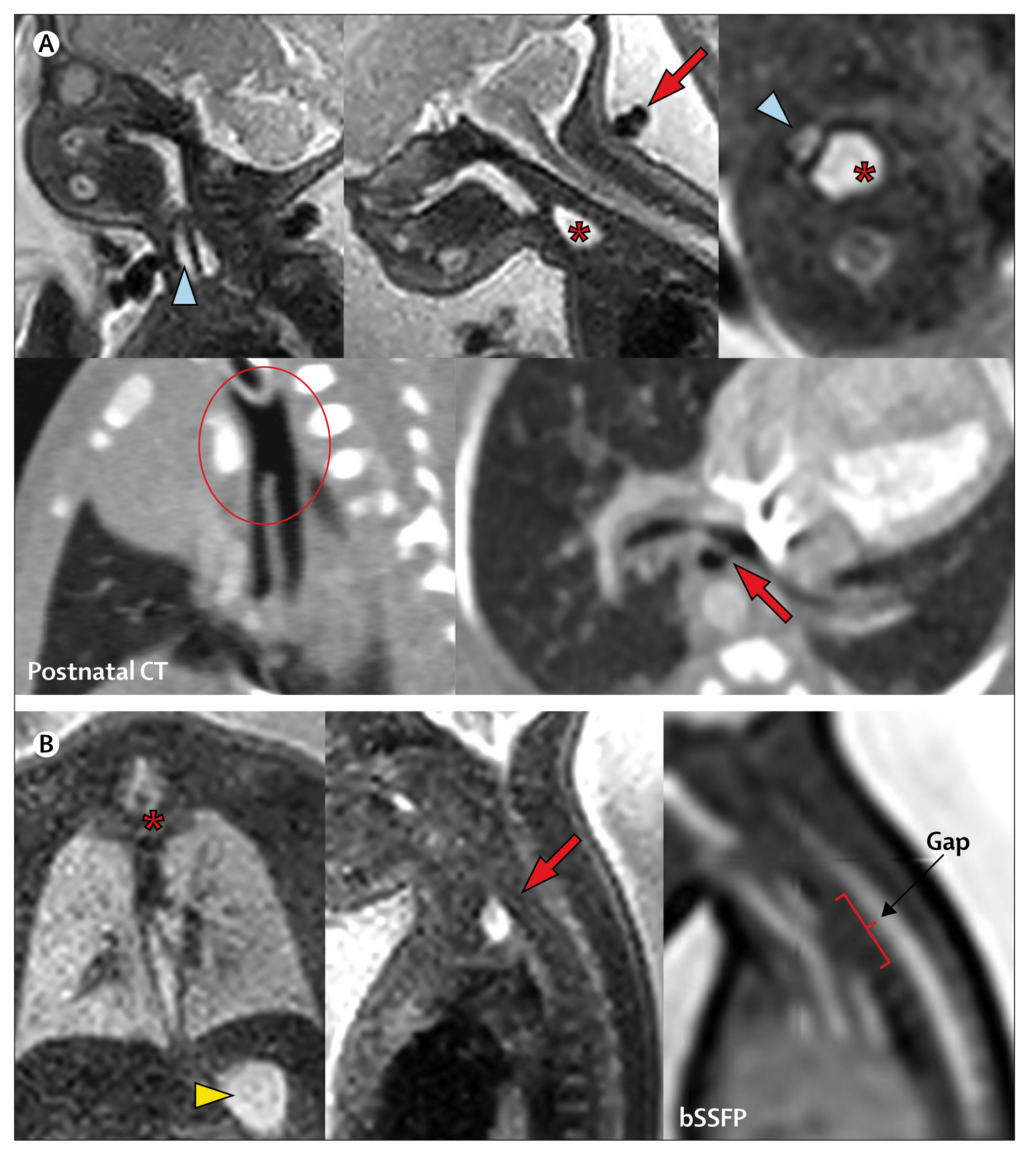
MRIs of two fetuses with oesophageal atresia (A) Fetus at 32 weeks and 5 days of gestation has a dilated upper pouch (marked by an asterisk), displacing the trachea (blue arrowhead) in the axial view (upper right image). A two-vessel umbilical cord (red arrow) can be seen in the sagittal plane (top middle image). The postnatal CT (lower two images) shows a long-segment common tracheoesophageal channel (encircled, left) and distal fistula arising from the left main bronchus (red arrow, right). (B) Fetus at 30 weeks and 5 days of gestation has a dilated upper pouch (marked by an asterisk in the left image and an arrow in the central image) and normal stomach (arrowhead), suggesting a distal fistula. Gap measurement is possible in the sagittal image (right). bSSFP=balanced steady-state free precession MRI.
